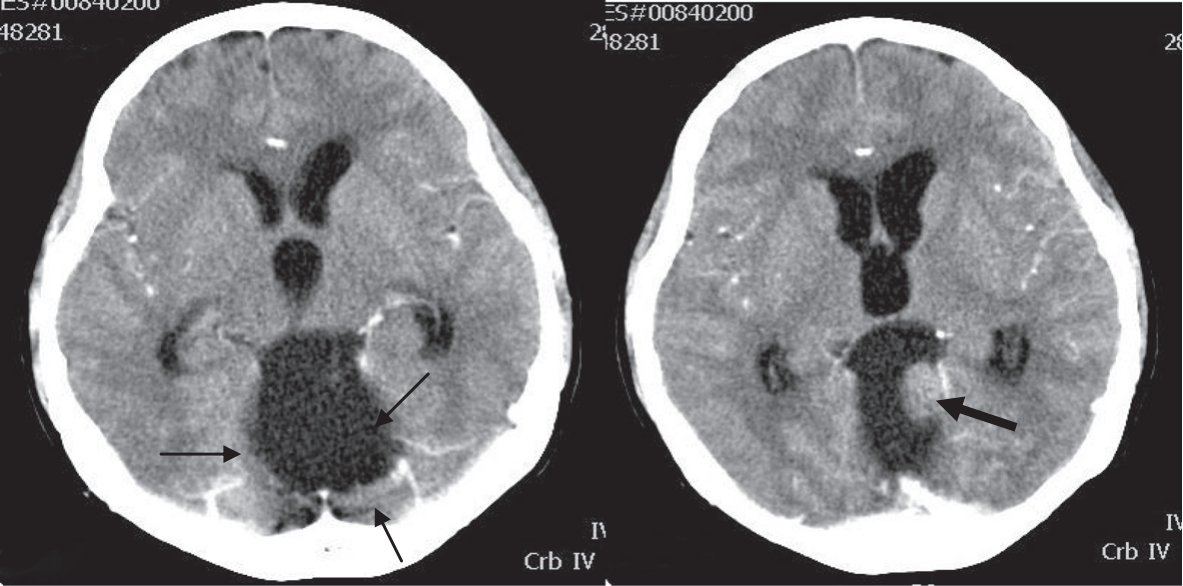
Axial contrast-enhanced computed tomography brain scan showing a large posterior fossa cystic mass (thin black arrows) with enhancing nodule (thick black arrow) compressing 4th ventricle causing obstructive hydrocephalus.
